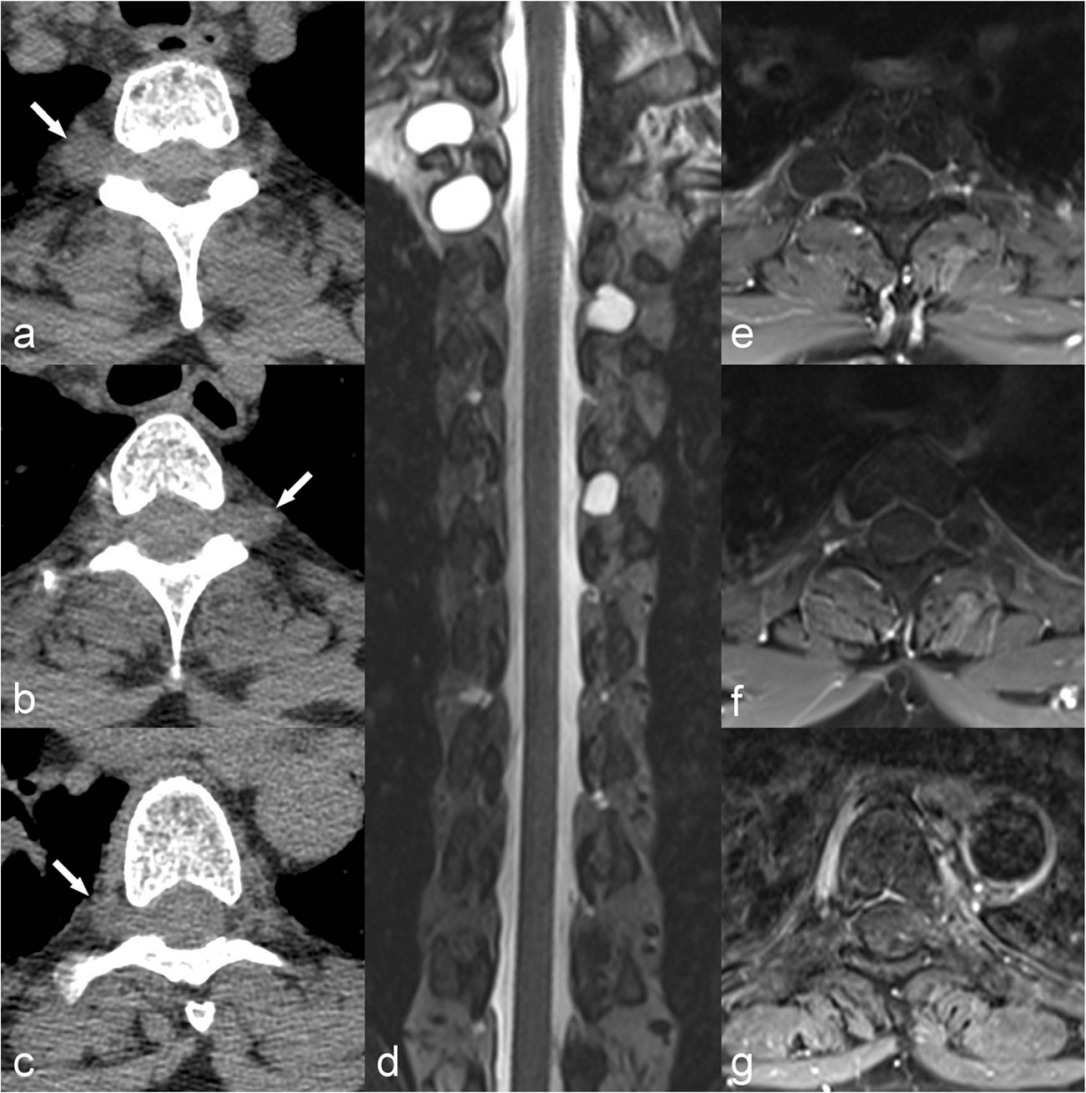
CT scan (a,b,c) performed in a 61-year-old females complaining of back pain after a fall shows several paraspinal lesions (arrows), which are difficult to characterise by this technique. A CISS MR sequence (d) clearly shows these to be extradural cysts. Note that there is no enhancement on T1 FS Gd (e,f,g)
